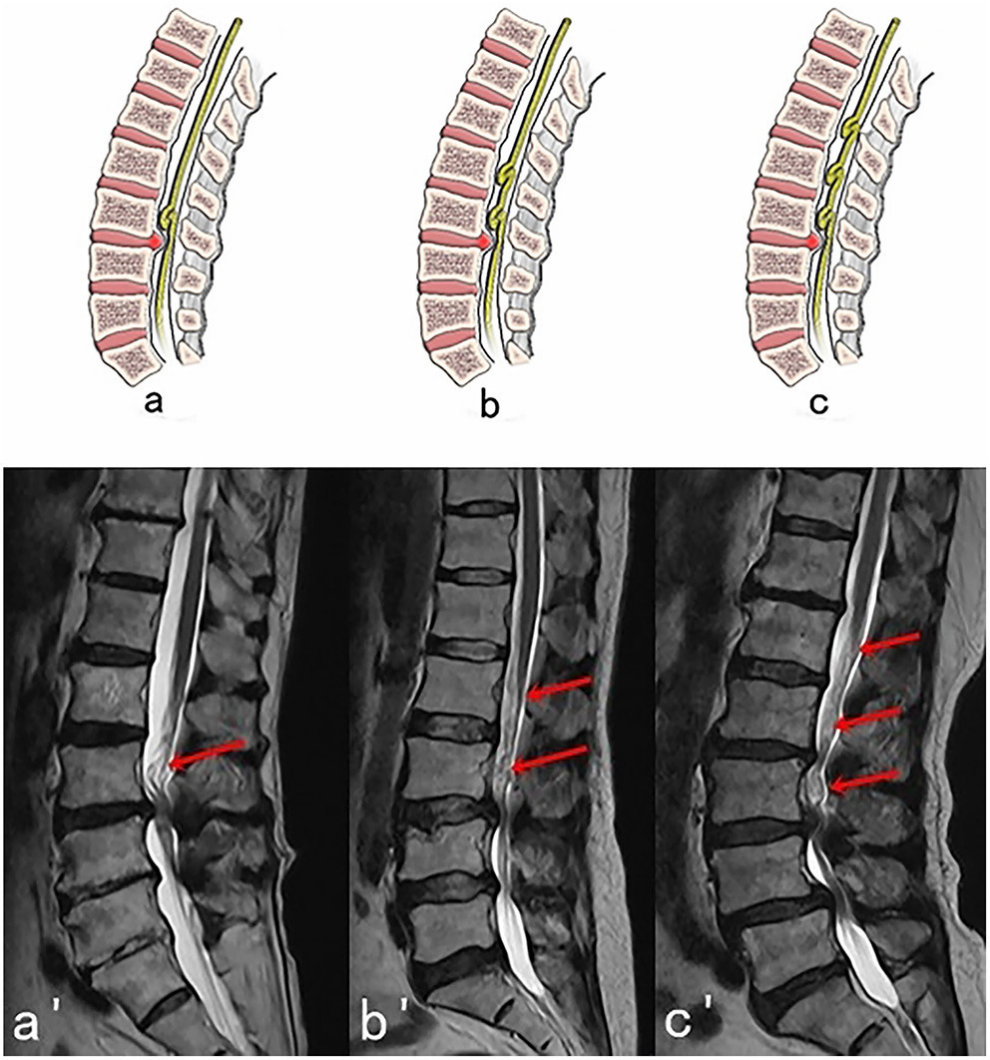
Three possible subtypes of Upper RNRs (RNRs which are above the stenosis) with corresponding MRI images. (A,A′) One segment of RNRs (red arrow) are above the stenosis. (B,B′) Two segments of RNRs (red arrows) are above the stenosis. (C,C′) Three segments of RNRs (red arrows) are above the stenosis.
